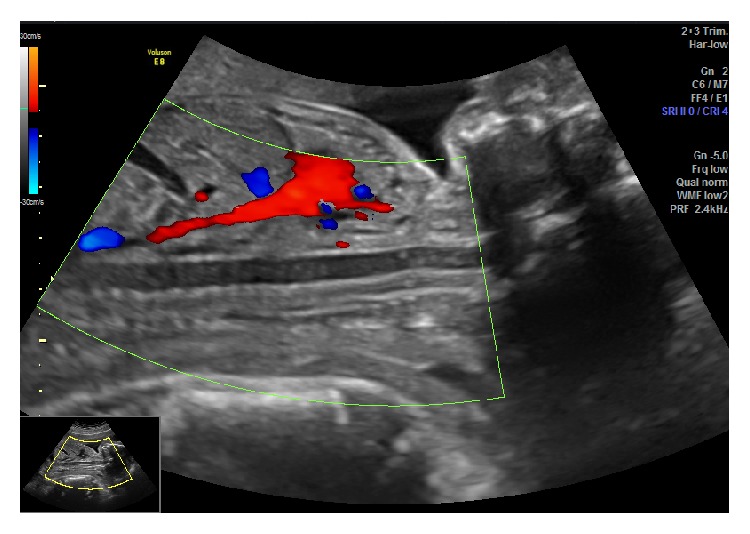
Color Doppler showing absence of flow through the notochord.
